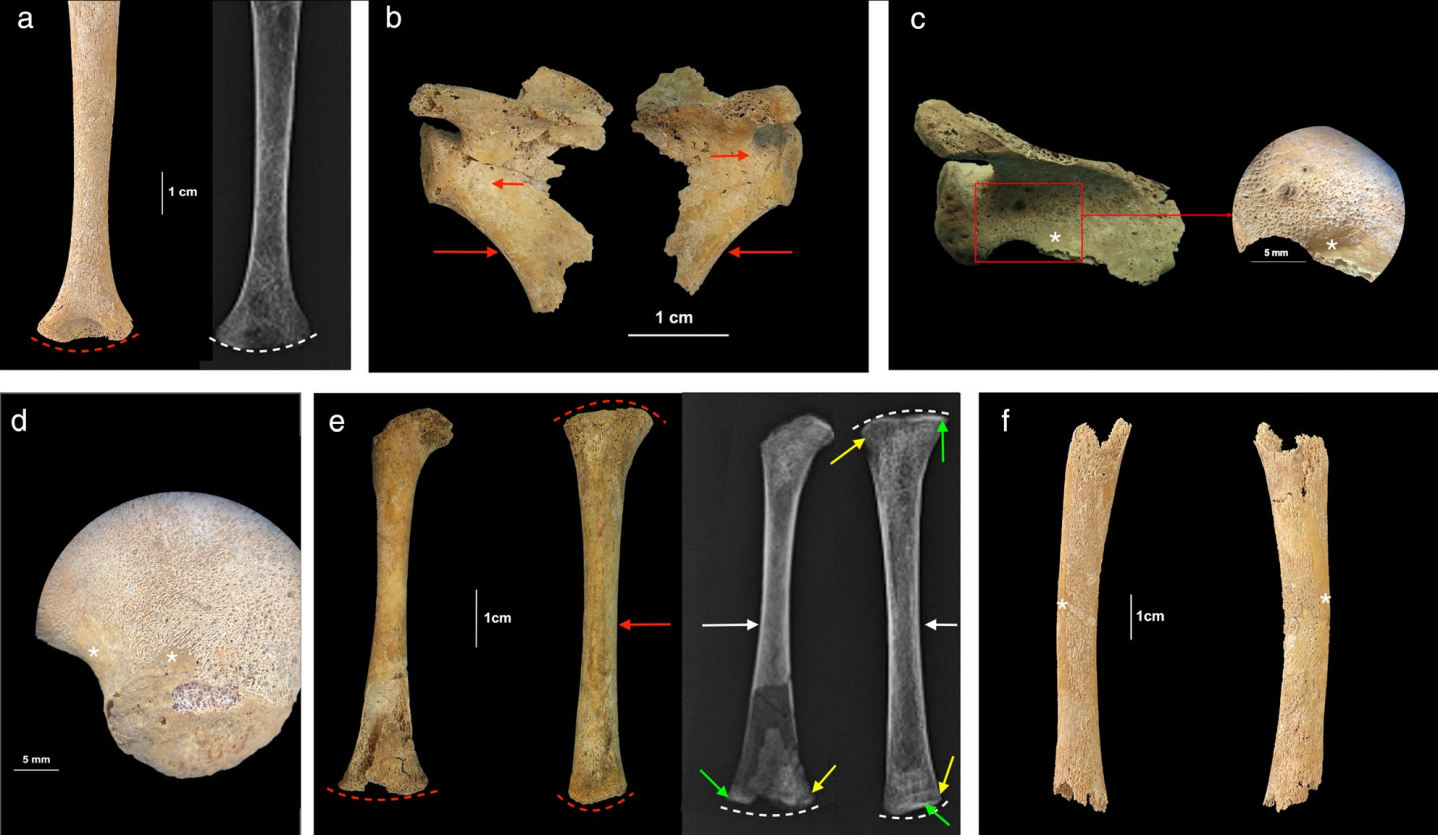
Individual PC4475 (1.5–2.5 years). (a) Posterior view of the right humerus with diffuse SPNBF and evidence of mild metaphyseal enlargement (red dashed line), also confirmed by x-ray (white dashed line); (b) dorsal view of the scapulae with bilateral abnormal porosities < 1 mm in diameter penetrating cortical bone of the *infraspinatus fossae* and SPNBF, also along both axillary margins (red arrows); (c) superior view of the right scapula with SPNBF on the *supraspinatus fossa* and close-up (red square) of the layer of new bone formation compared to the original cortex (*); (d) gluteal surface of the right ilium showing diffuse SPNBF attached to the original cortex (*); (e) anterior view of the right femur and tibia showing distal metaphyseal enlargement of the former and enlargement of both ends in the latter (red dashed lines). The tibia exhibited diffuse SPNBF on the medial side (red arrow). X-ray confirmed the metaphyseal defects (white dashed lines) and revealed distal Fränkel white lines (green arrows) and ‘scurvy lines’ (or Trümmerfeld zone) in the right femur and in both extremities of the tibia (yellow arrows). The presence of ‘pencil thin cortex’ of the femoral diaphysis (white arrow) was also radiologically visible as well as the presence of pathological SPNBF of the tibia (white arrow). Generalized ground-glass appearance of the long bones was visible with osteopenia in the proximal tibia; (f) Lateral (left side) and medial (right side) surface of the left tibia preserved in its diaphysis showing SPNBF attached to the original cortex (*). Photo R.S. and G.R.
